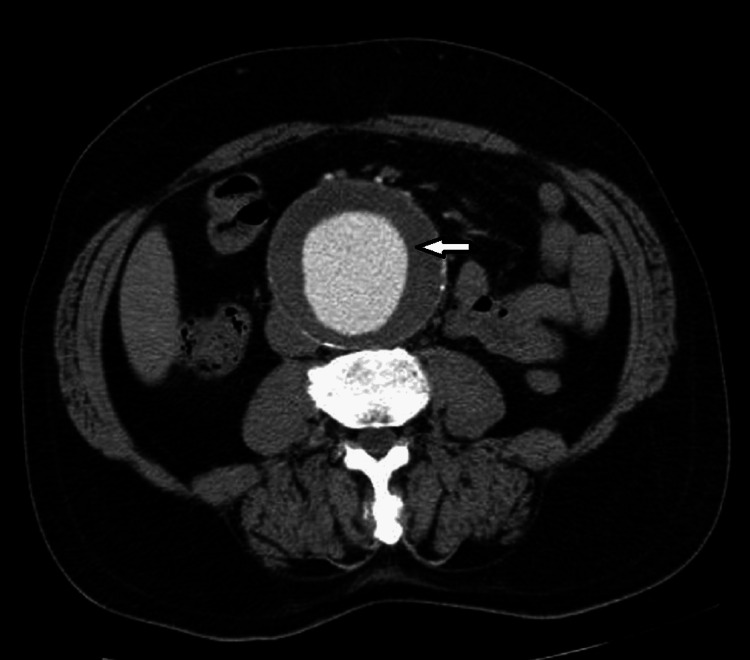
Axial contrast-enhanced computed tomography revealing a significant aneurysmal dilation originating from the posterior of the abdominal aorta below the renal arteries
